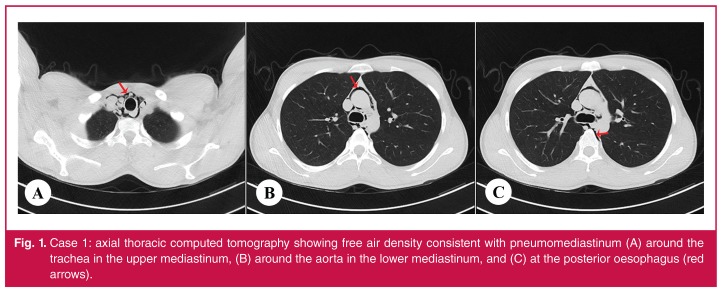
Case 1: axial thoracic computed tomography showing free air density consistent with pneumomediastinum (A) around the trachea in the upper mediastinum, (B) around the aorta in the lower mediastinum, and (C) at the posterior oesophagus (red arrows).
